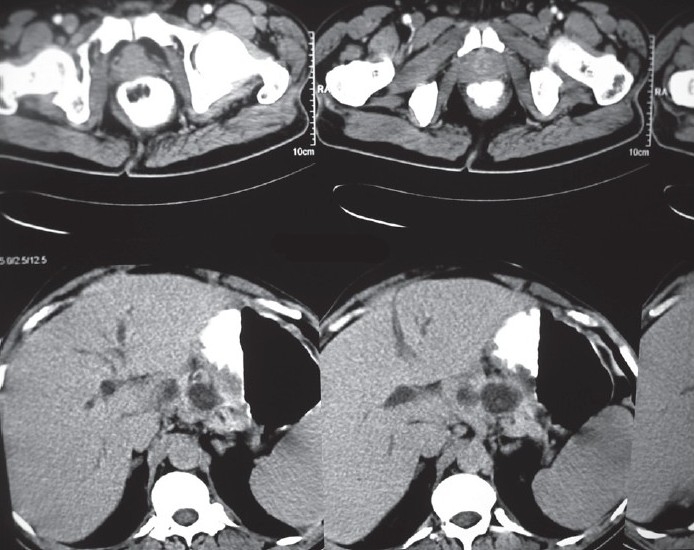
CT scan of the pancreas showing pseudocysts in the body and tail.
